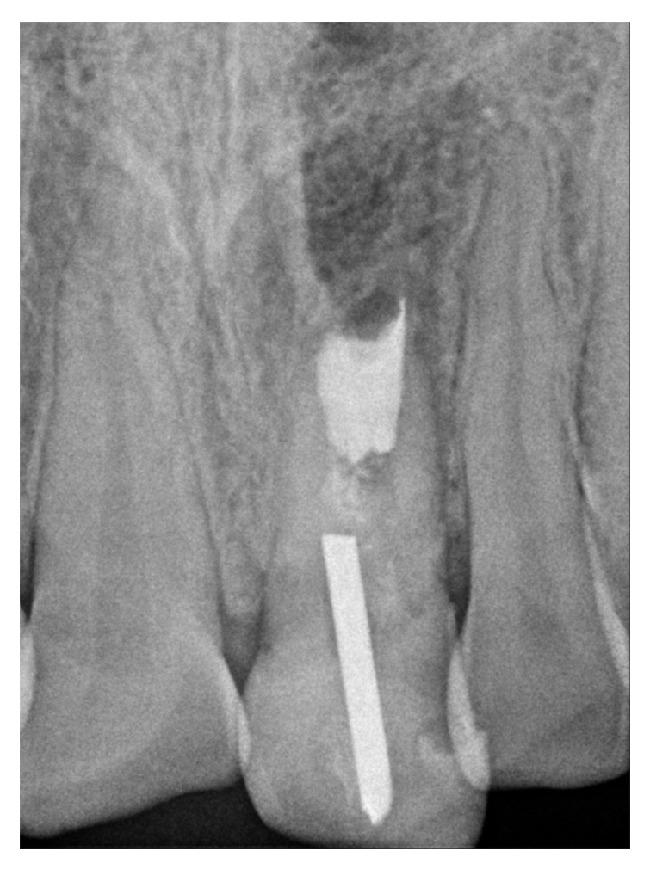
A follow-up radiograph at 12 months reveals a marked decrease of the periapical lesion, which was in the process of healing.
